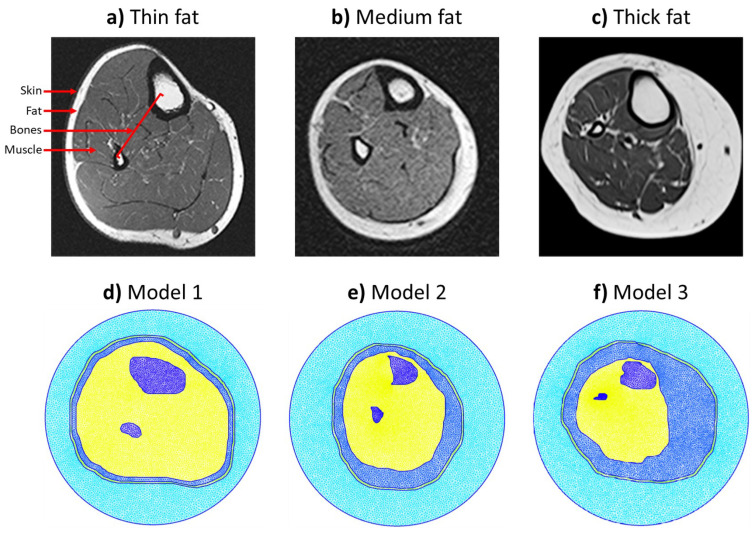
Generation of the three cross-sectional MRI-based anatomically-realistic leg models representing three fat thickness scenarios: (a,d) Thin fat model 1 [27], (b,e) Medium fat model 2 [28], and (c,f) Thick fat model 3 [29].
